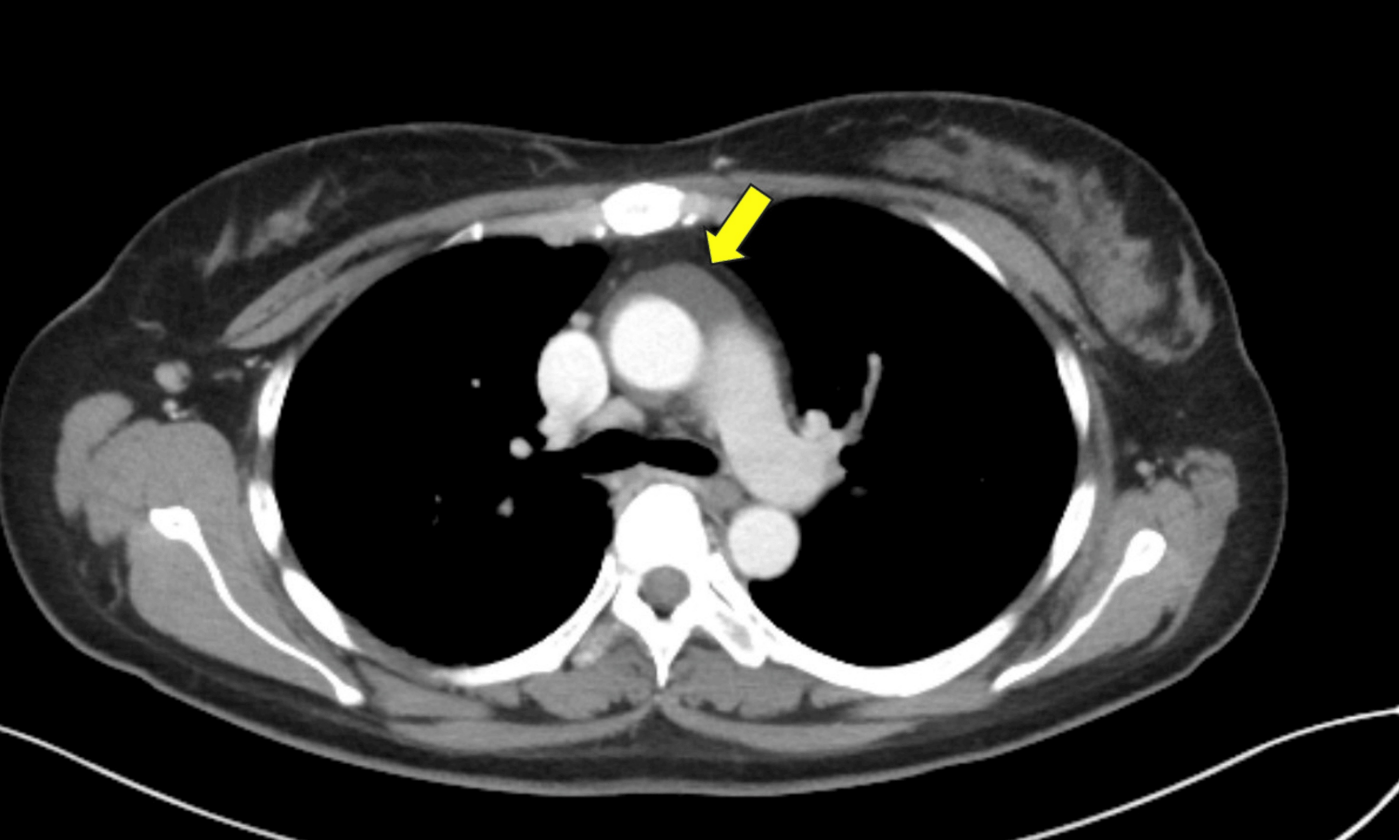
Contrast-enhanced CT Apparent wall thickening was observed at the base of the ascending aorta (arrow). CT: computed tomography
